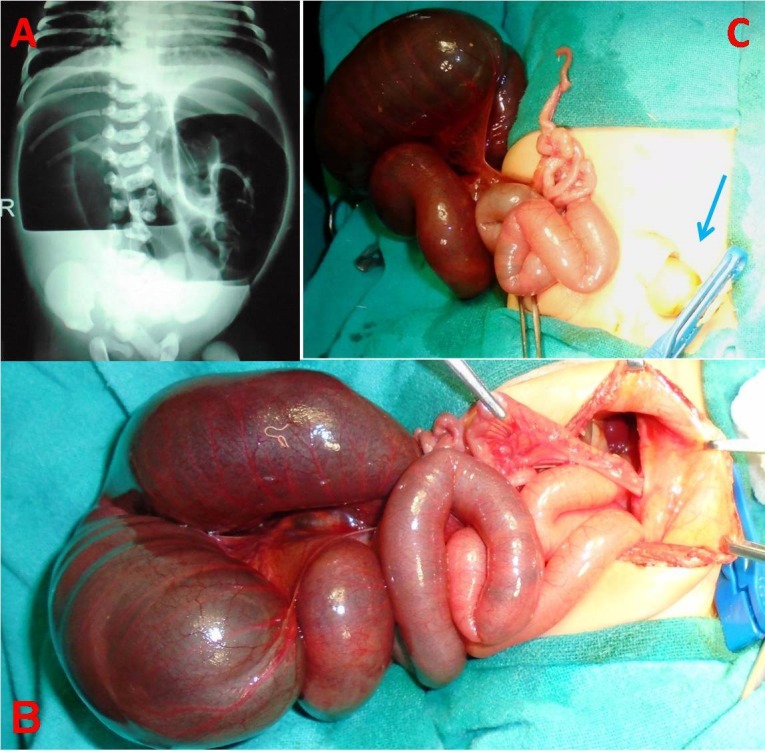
Figure 1: Upright abdominal radiograph showing dilated small bowel loops, multiple air-fluid levels with absence of air distally (A); Intra-operative picture showing type IIIa ileal atresia with distal unused ileum is seen herniating into the umbilical cord (B); hernia of umbilical cord (blue arrow) is seen along with proximal part of distal ileum after its dissection from inside the sac (C).
